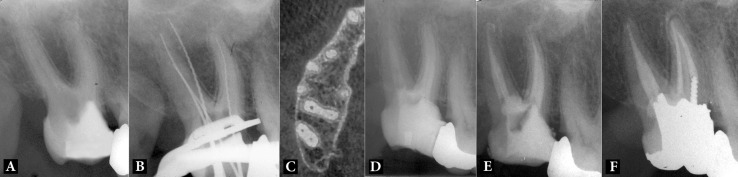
A*) Pre-operative radiograph, *B*) Perforation of the chamber floor was confirmed, *C*) CBCT of the respective area, *D*) Repair of the perforation site and intra-canal calcium hydroxide medicament, *E*) Post obturation radiography showing four canals, *F*) One-year follow-up
